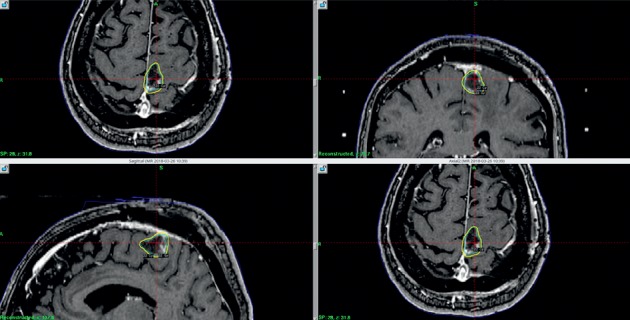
Multiple views of the Gamma Knife treatment plan with 22 Gy prescribed to the periphery of the tumor cavity, and this correlates with the 50% isodose line (dose representations are overlayed on a brain MRI from the day of treatment).
